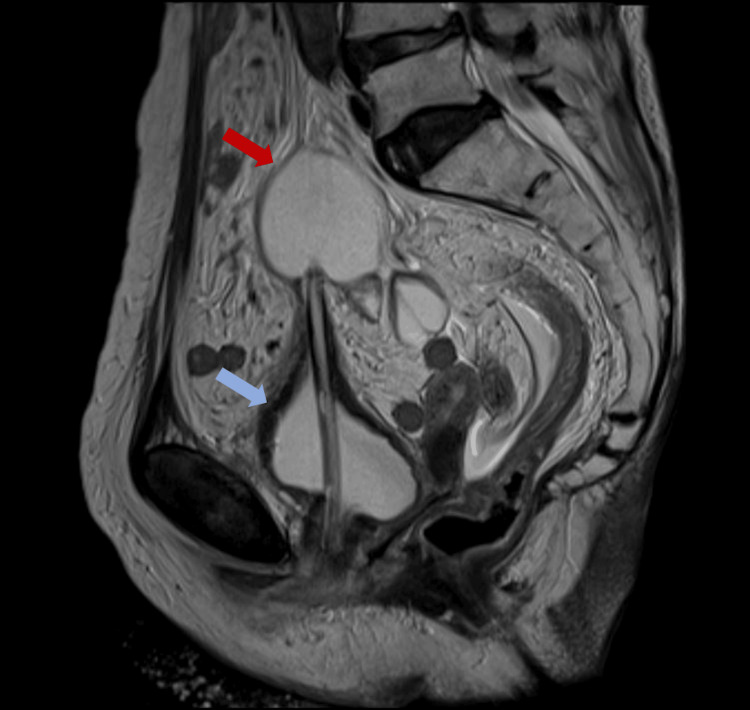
Sagittal view in MRI of the bladder perforation. The red arrow points to the catheter balloon; the blue arrow points to the deformed bladder.
